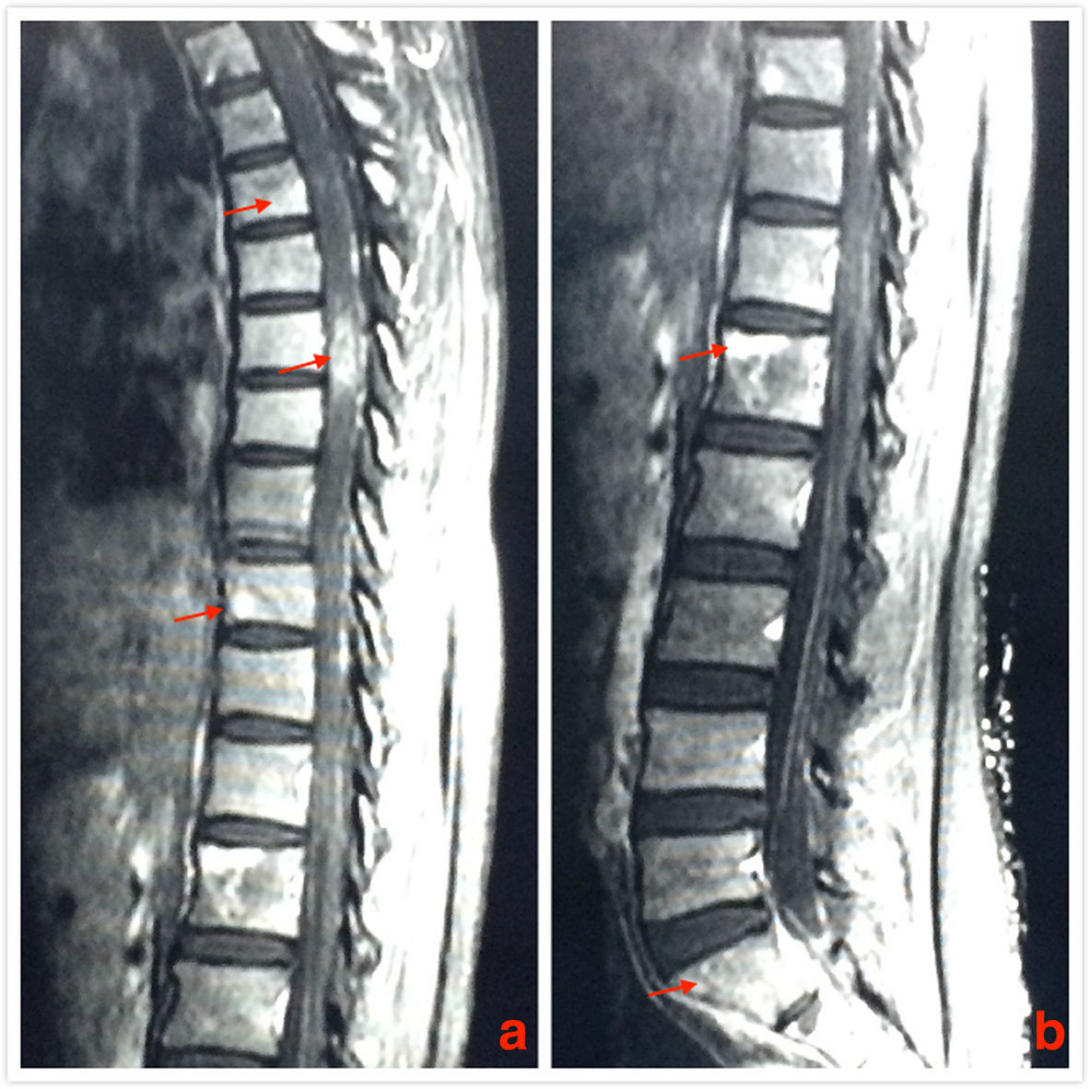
Spinal vertebral MRI confirming multiple metastases in the thoracic (a) and lumbar (b)
